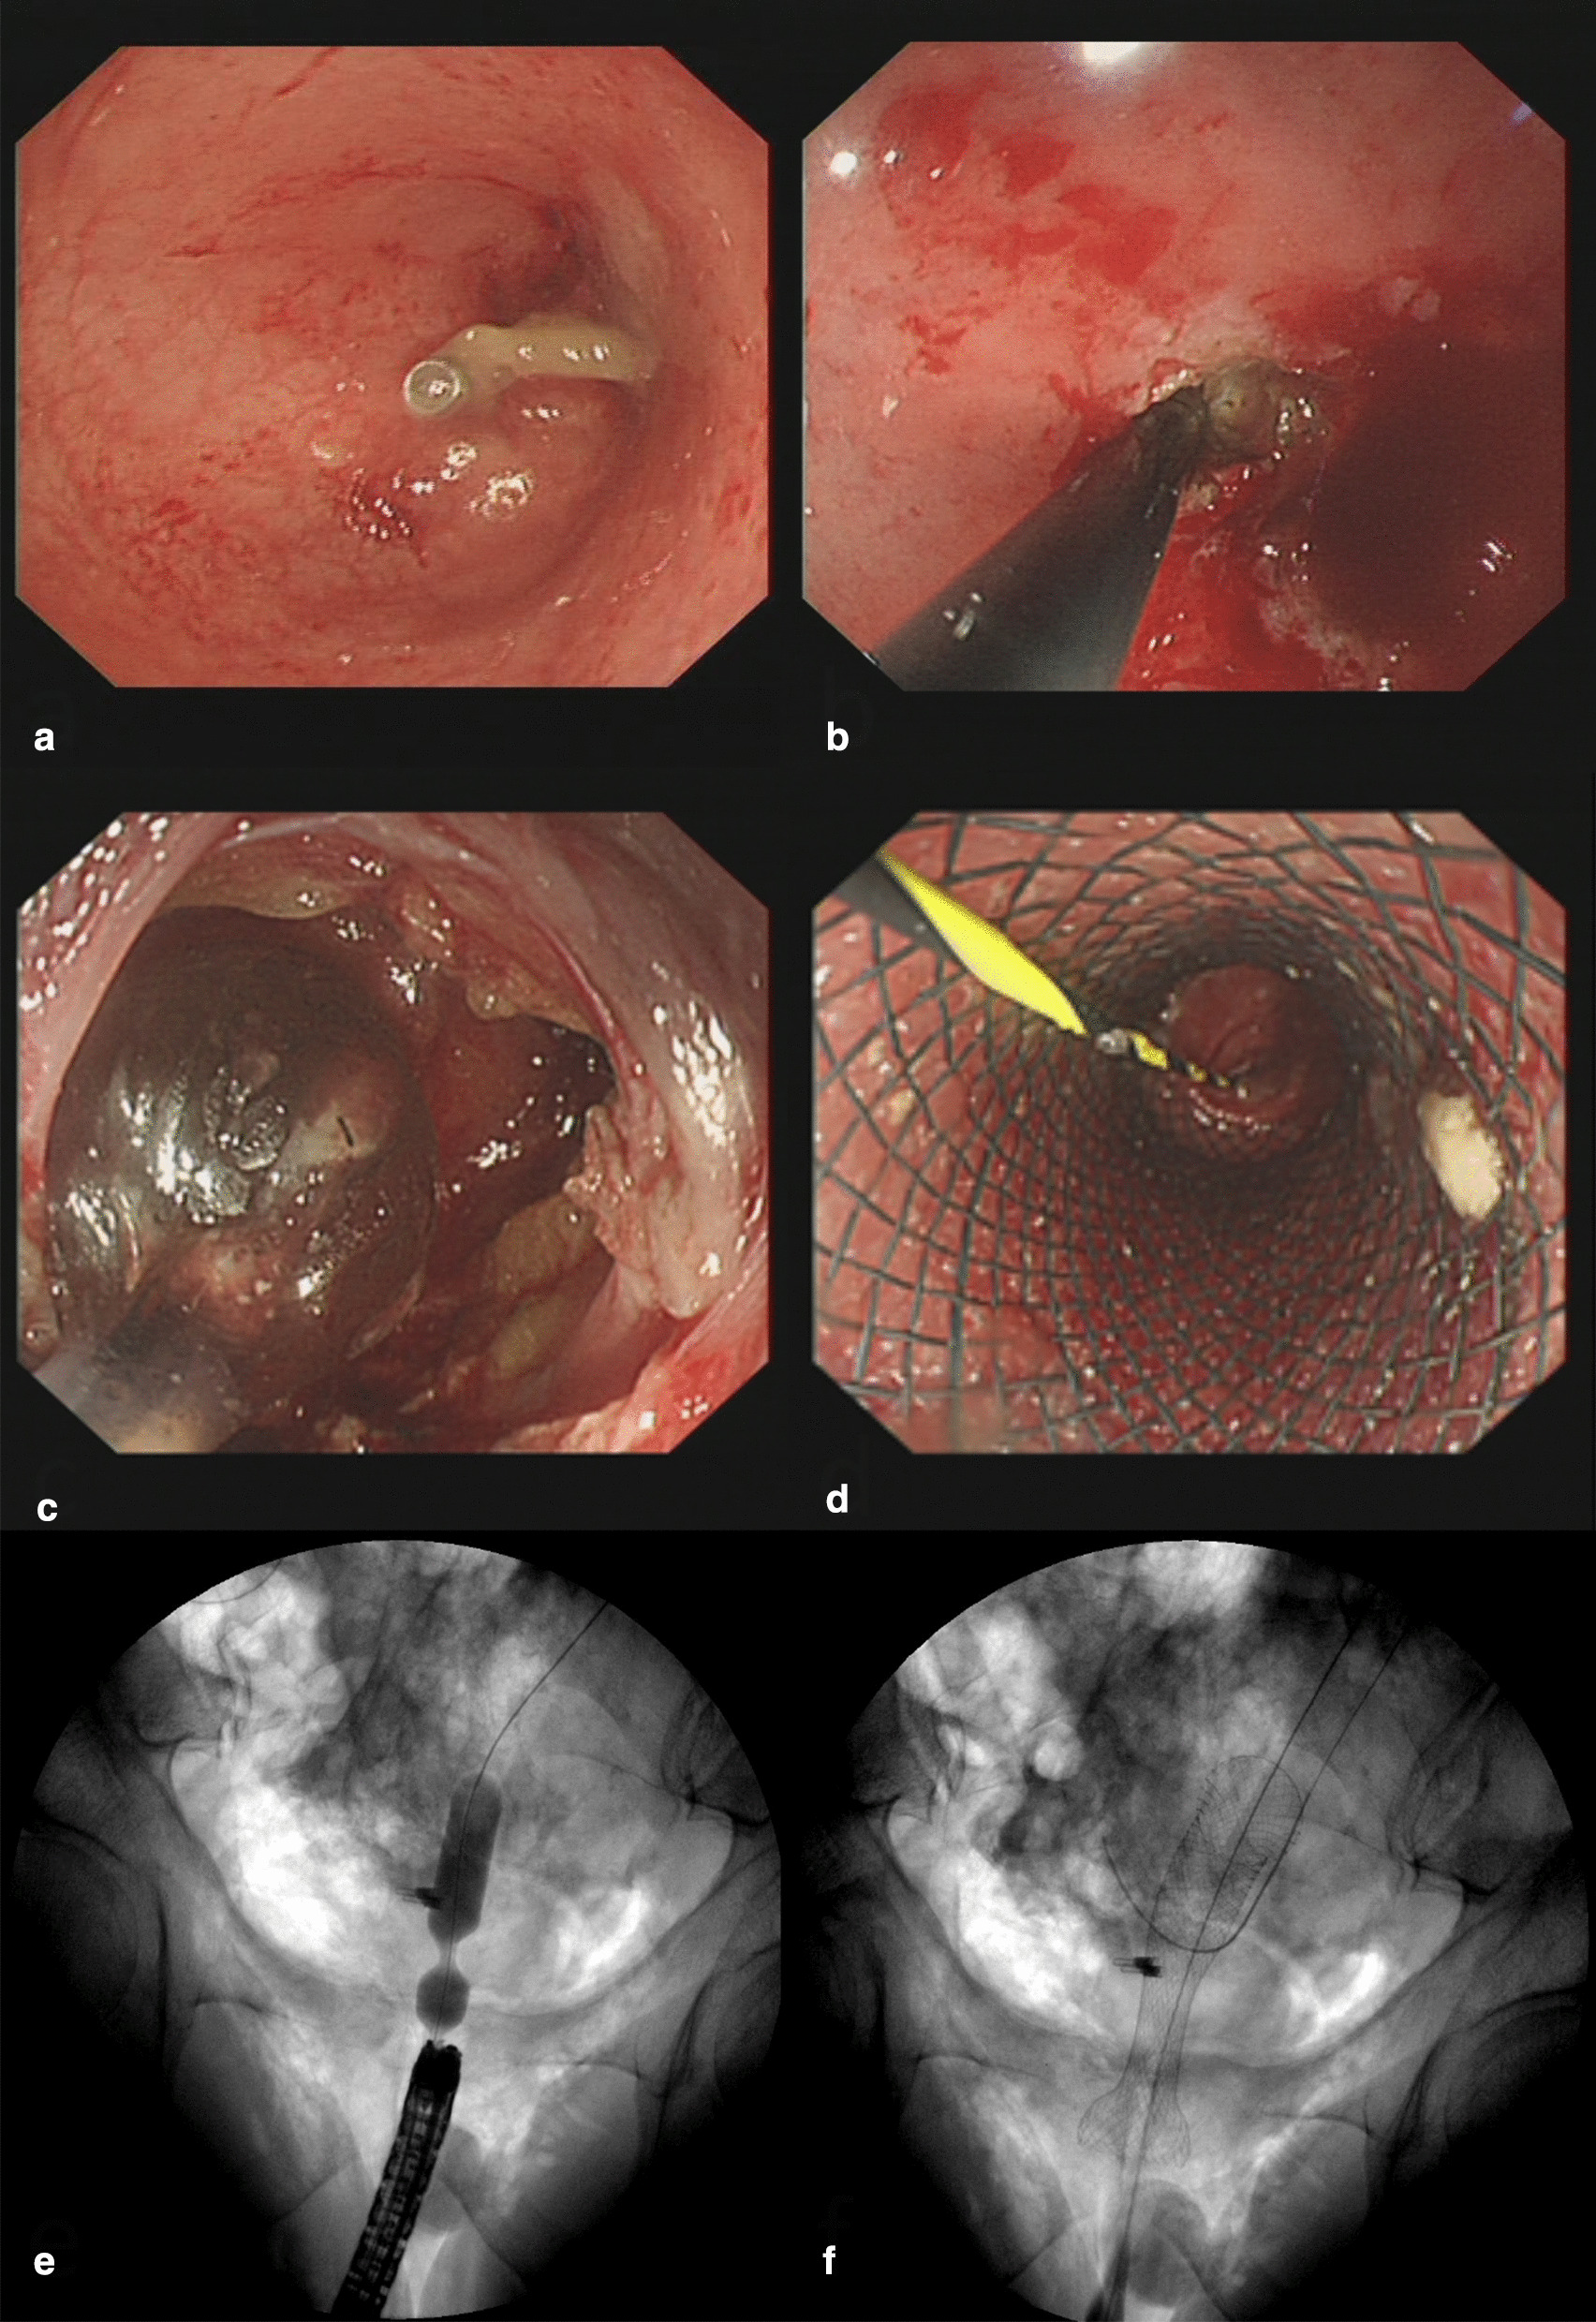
The patient's first endoscopic surgery procedure. a Titanium clips were labeled at the blind end of the proximal intestine. b The center of the blind end of the intestine was cut layer by layer with a hook knife. c Endoscopic application of dilating tubes and dilating balloons was performed to dilate the incised blind end of the intestinal canal. d The 60 mm * 20 mm self-expanding covered stent was inserted over the guide wire to bridge the two sides of the intestine. e On X-ray fluoroscopy, the dilated balloon was observed to gradually dilate the narrow blind end of the intestine. f Smoothly placed SEMS for dilation and bridging of the intestinal tube was visible under X-ray fluoroscopy
